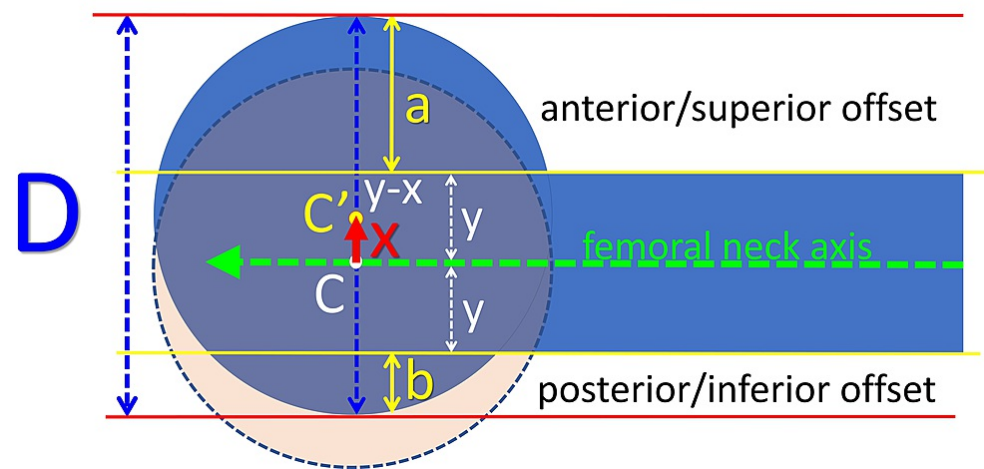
Calculation of the center-axis distance. C and C': The center of the femoral head before and after slippage, respectively; D: the diameter of the femoral head (blue-dotted arrow); green arrow: the femoral neck axis; a: anterior/superior head-neck offset on the frog-lateral/anteroposterior pelvis x-ray, respectively; b: posterior/inferior head-neck offset on the frog-lateral/anteroposterior pelvis x-ray, respectively; y: the radius of the femoral neck; x: the center-axis distance (CAD, red arrow).
